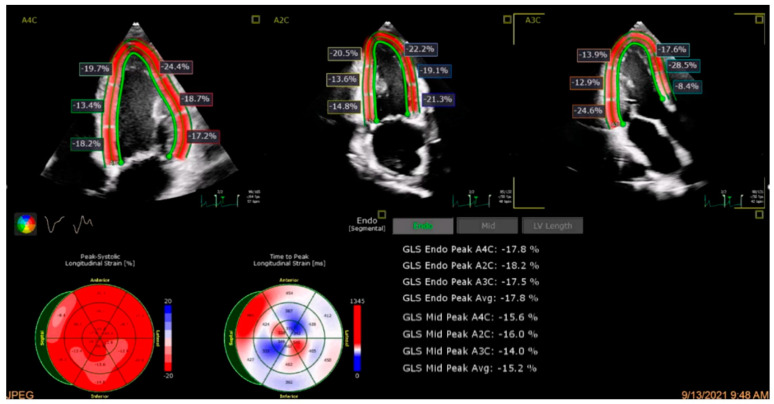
Example of artificial intelligence model to automate global longitudinal strain (GLS) on transthoracic echocardiogram. Through automated contouring of endocardial and epicardial borders on apical 4-chamber (A4C), apical 2-chamber (A2C), and apical 3-chamber (A3C) views, generation of peak endocardial and mid strain is presented.
